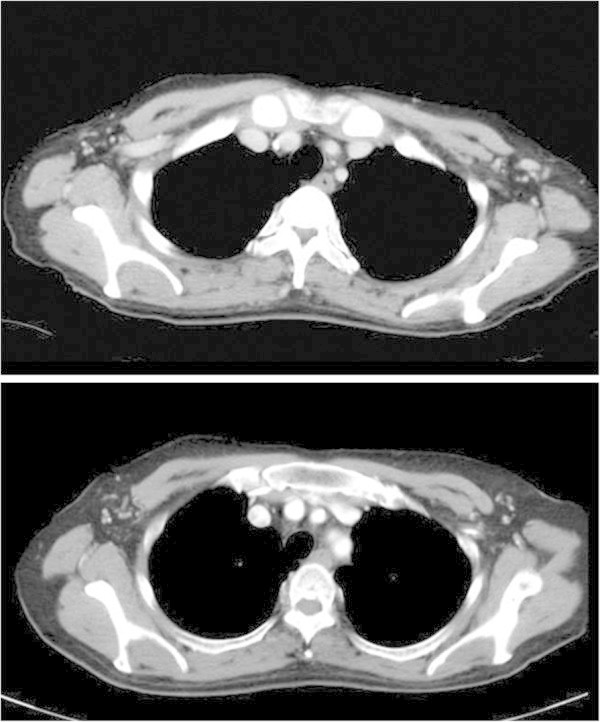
CT findings of the bilateral axillary lymph nodes at pre-and post-treatment. At the start of treatment, several lymph nodes in the left axilla were clearly swelling indicating metastasis (upper). After neoadjuvant chemotherapy those were not swelling (lower).
